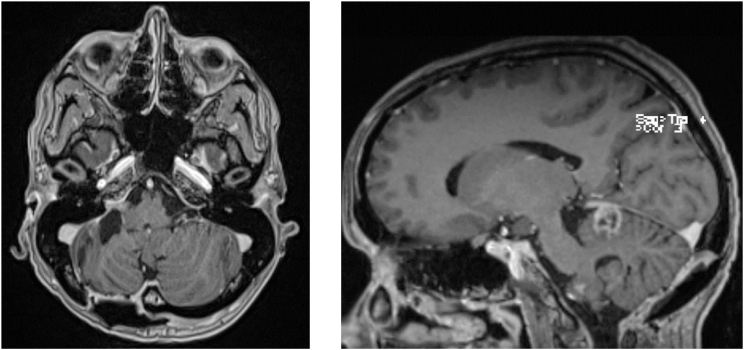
Postpartal MRI T1 with contrast depicting stable tumor at the resection cavity (left) and a second infratentorial lesion (right).
